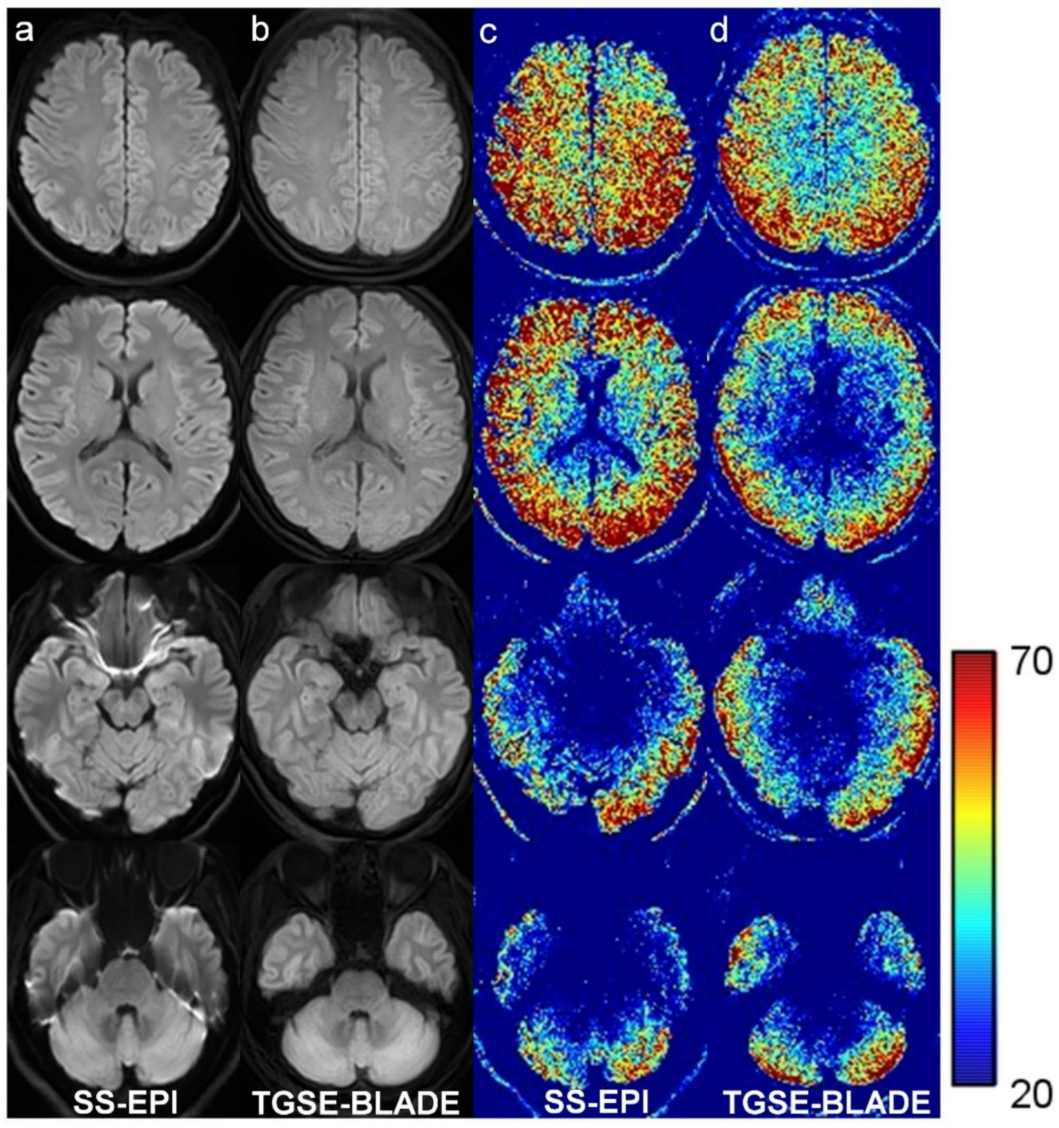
Representative b1000 images (a, b) and SNR maps (c, d) of SS-EPI DWI (a, c) and TGSE-BLADE DWI (b, d) are shown in a healthy volunteer.
